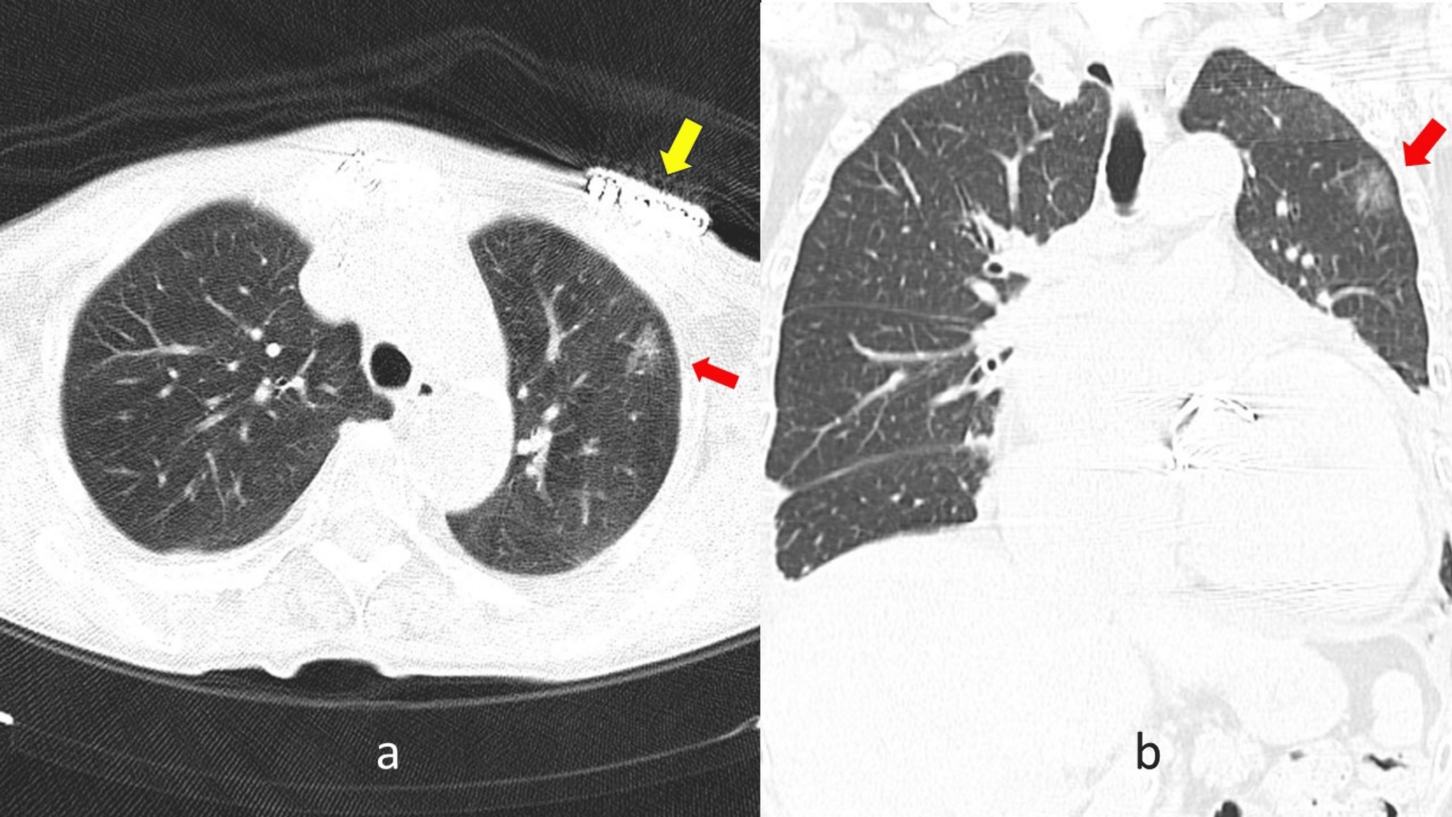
CT scan films before operation. Red arrows show ground glass opacity just behind the pacemaker. The yellow arrow shows the implanted pacemaker. (a) Cross-sectional view and (b) frontal view.
